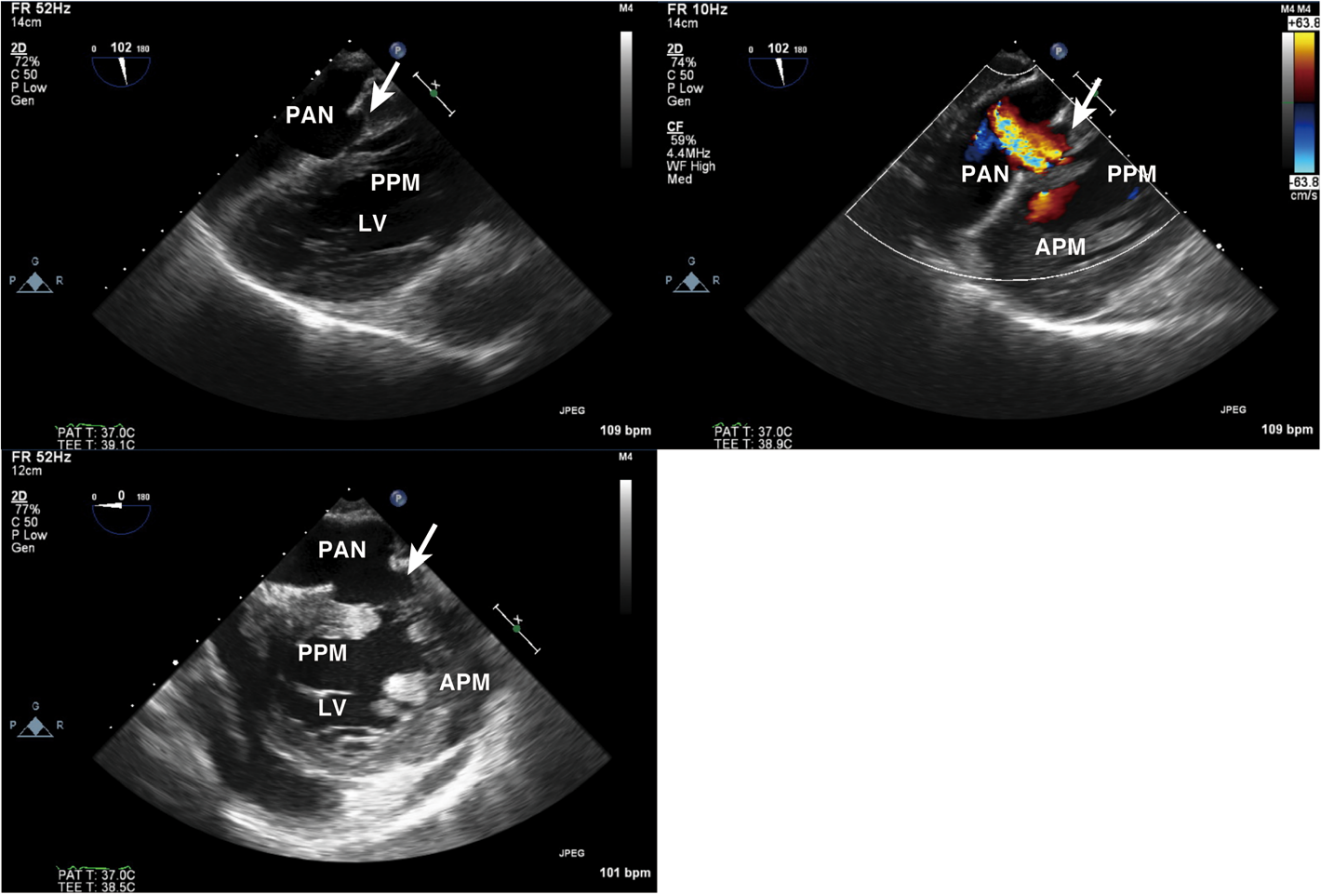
Transgastric long-axis view (two-dimensional, upper left; color flow Doppler, upper right) and mid-papillary short-axis view (lower). The orifice of the pseudoaneurysm is located in the inferior wall of the left ventricle (arrow). APM anterolateral papillary muscle, LV left ventricle, PAN pseudoaneurysm, PPM posteromedial papillary muscle
